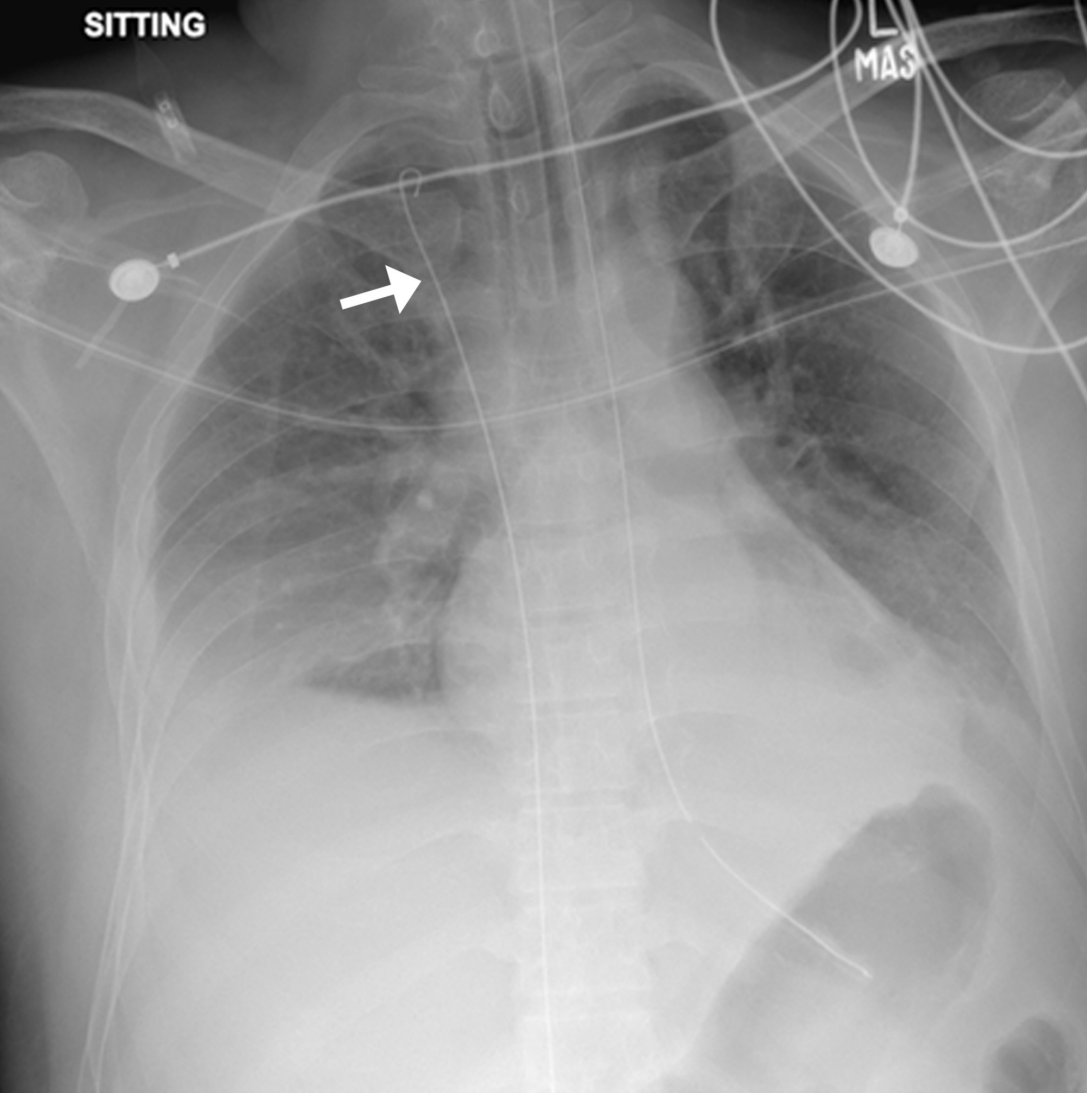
Post-procedural portable chest radiograph showing trapped guidewire (arrow); nasogastric tube and endotracheal tube also seen in place
